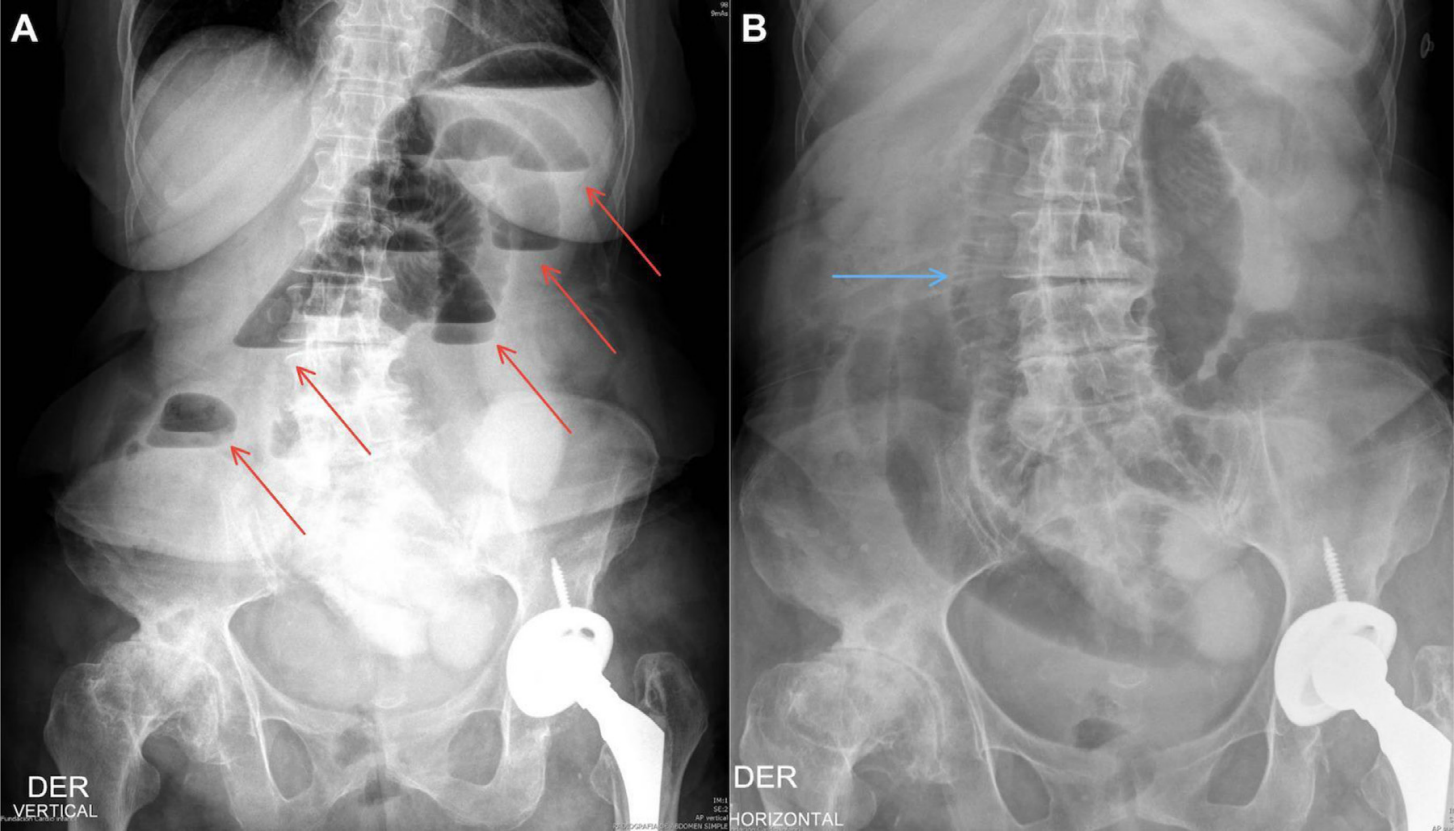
Abdomen radiographs in frontal projection in an upright position (A) and supine position (B). Distended small bowel loops with step-ladder air-fluid levels (red arrows) and thickened valvulae conniventes (blue arrow) are observed, findings indicative of small bowel obstruction. Additionally, severe degenerative changes are noted in the right hip joint, along with a total hip replacement on the left side.
